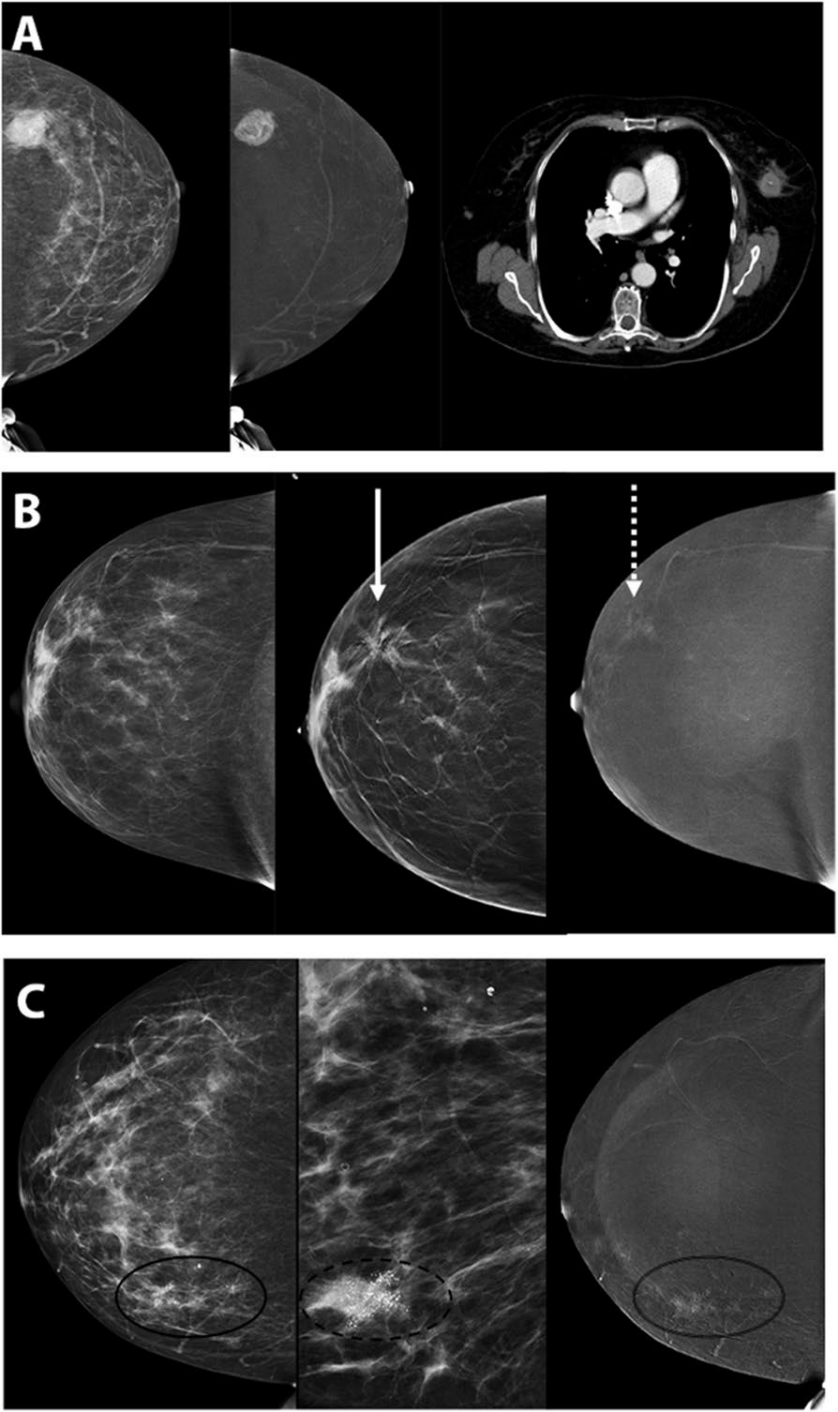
High Risk Lesions or Borderline. A 76-year-old woman presented with new hyperdense mass in left upper outer breast on LE images, with avid enhancement and central necrosis on RI CEM. Patient unable to undergo MRI due to pacemaker but subsequent staging CT chest image shows a corresponding mass. Patient underwent left mastectomy which revealed malignant phyllodes tumor. B 40-year-old woman presenting for baseline screening mammogram, called back for architectural distortion. On CEM diagnostic workup, there is persistence of the architectural distortion (solid arrow) on LE images with minimal central enhancement (dash arrow). Ultrasound (not pictured) revealed a subtle area of distortion. Subsequent biopsy was compatible with radial scar, atypical ductal hyperplasia, intraductal papilloma. C Patient presented with suspicious microcalcifications (solid circle on LE image, dash circle on magnified view, and double line circle corresponding to non-mass enhancement on RI)
